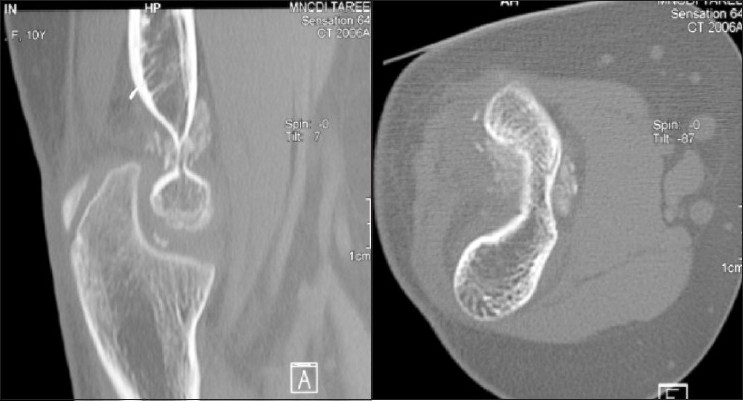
CT scan of the left elbow showing demineralisation of bones in distal humerus, radius and ulna, with disrupted appearance of trochlea. Bone fragments are seen both anteriorly and posteriorly
